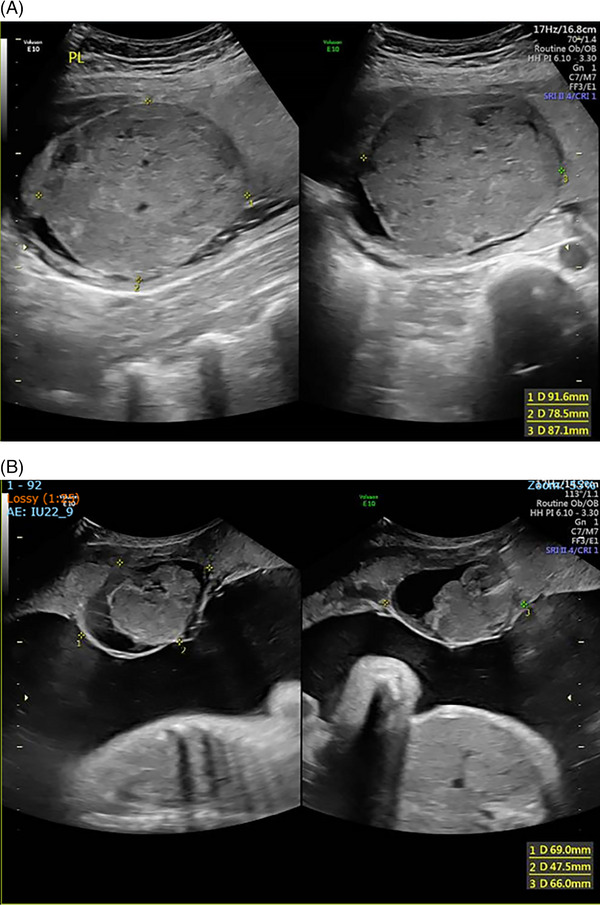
(A) Sonographic appearance of a giant placental chorangioma. Note the relatively homogeneous echotexture. (B) Giant chorangioma with a heterogeneous echotexture.
